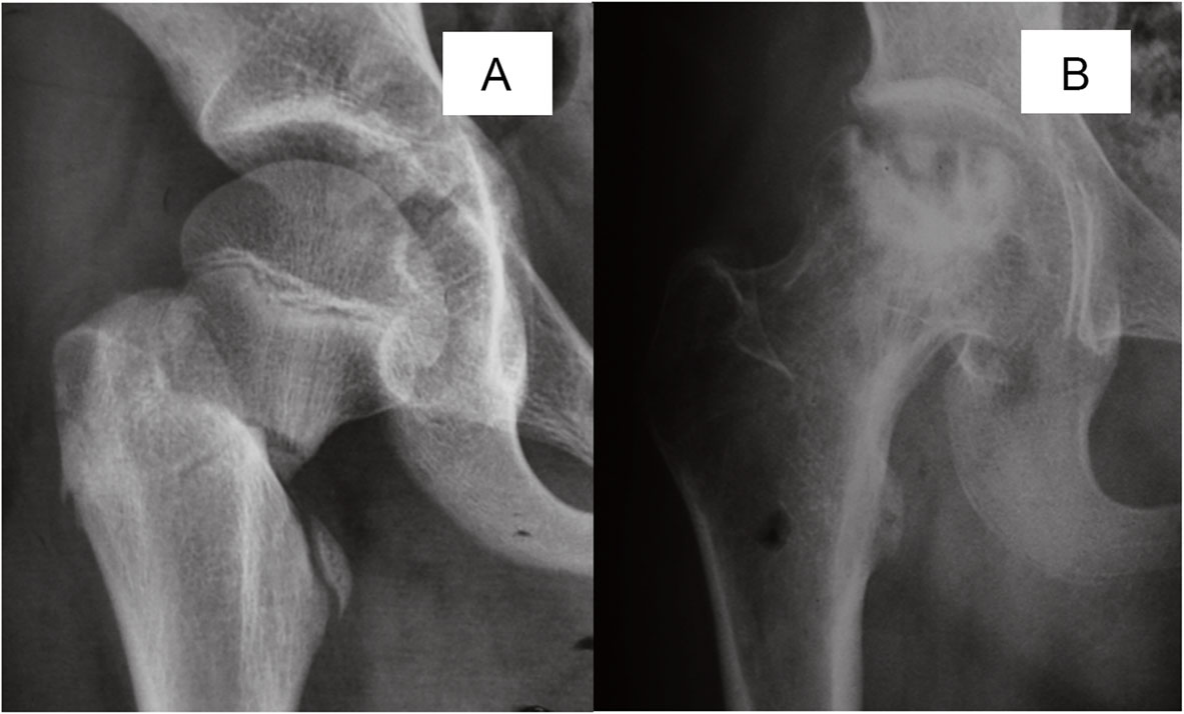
The anteroposterior radiographs of Ratliff type I avascular necrosis after pediatric femoral neck fracture. Femoral neck fracture occurred at age of 12 years (a) and avascular necrosis were diagnosed 8 months later (b), and JIC type C2, presenting collapsed femoral head
